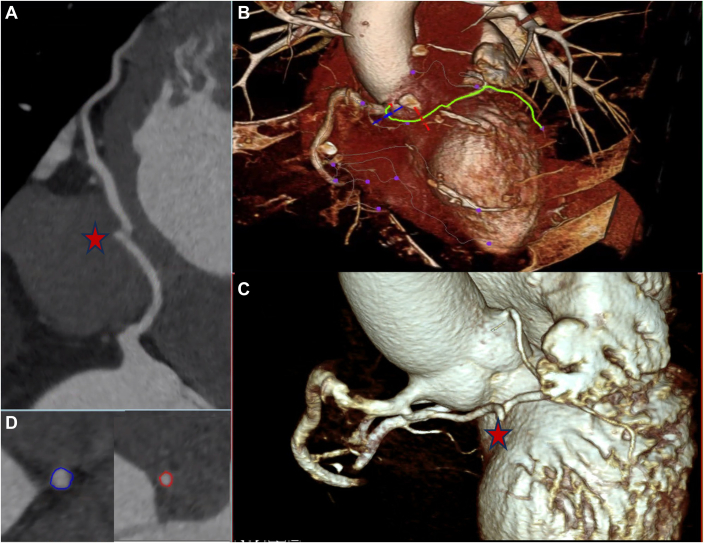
Computed Tomography Angiography of the Coronary Arteries (A) Coronary CT with curved multiplanar reformat of the anomalous coronary artery demonstrating a long intramyocardial course with a characteristic “curly-Q” configuration (red star). (B and C) Three-dimensional volume-rendered images of the coronary anatomy, shown with and without the right ventricular outflow tract for spatial reference. (D) Cross-sectional views of the arterial lumen highlighting significant narrowing (red) within the intramyocardial segment compared to the proximal segment (blue).
